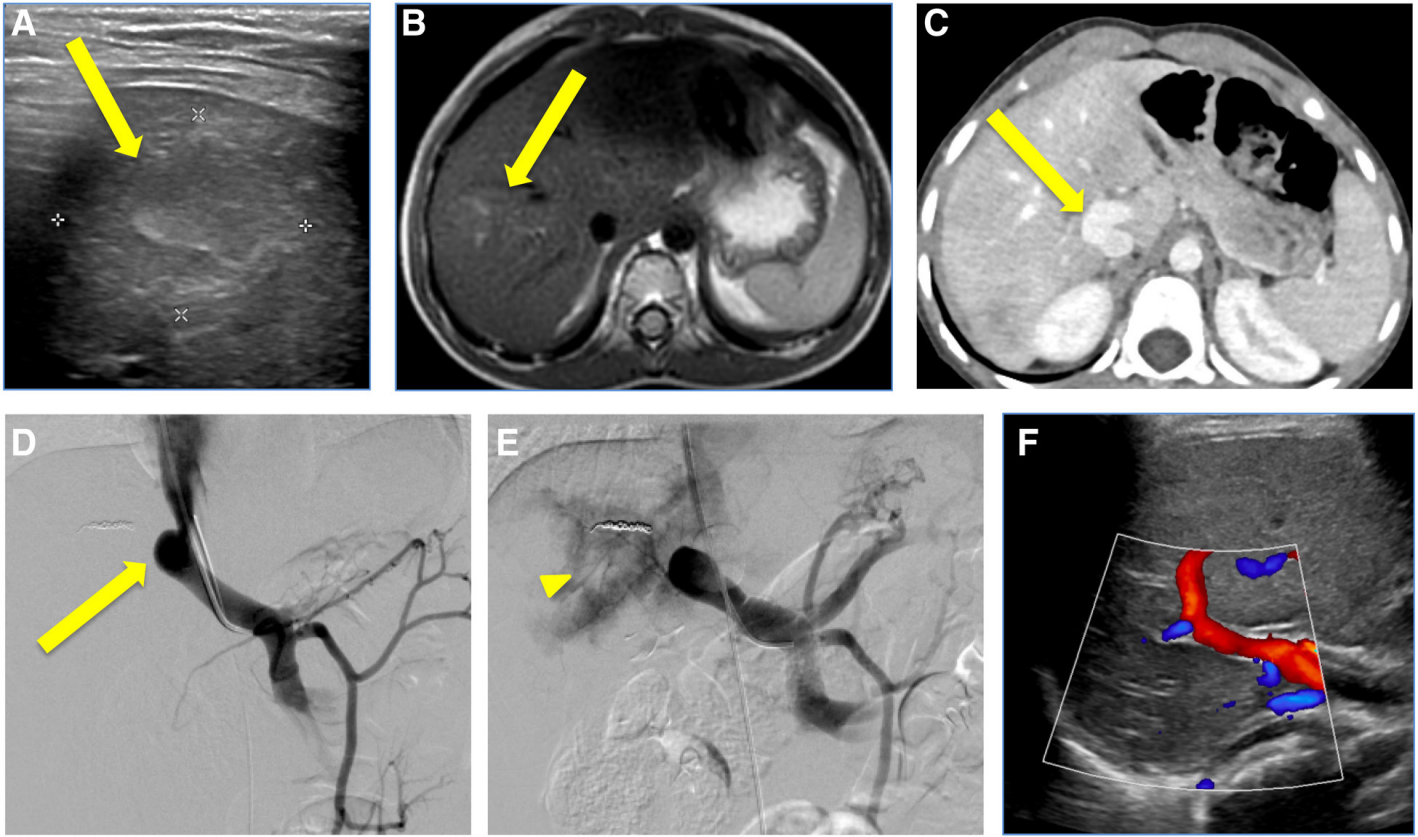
Preprocedure imaging, Interventional Radiology test occlusion venograms and post surgical follow-up imaging of the portosystemic shunt. A) Ultrasound showed an ill-defined lesion in the right lobe (arrow). B) MRI confirmed an indistinct right lobe lesion however the enhancement pattern was not specific for a benign or malignant lesion (arrow). C) CT showed an extrahepatic shunt (arrow) with a small left portal vein and a definitive right portal vein could not be detected. D, E) Venograms demonstrated a short and wide portosystemic shunt (arrow) from the diminutive left portal vein which drained directly into the inferior vena cava. Occlusion venogram showed redirected blood flow to the liver and filling of portal vein radicals (arrowhead). However the gradient was elevated and the morphology was favorable for surgical closure. F) Follow-up images show no signs of portal hypertension with good hepatopetal portal flow and resolution of the liver tumors. CT = computerized tomography; MRI = magnetic resonance imaging.
